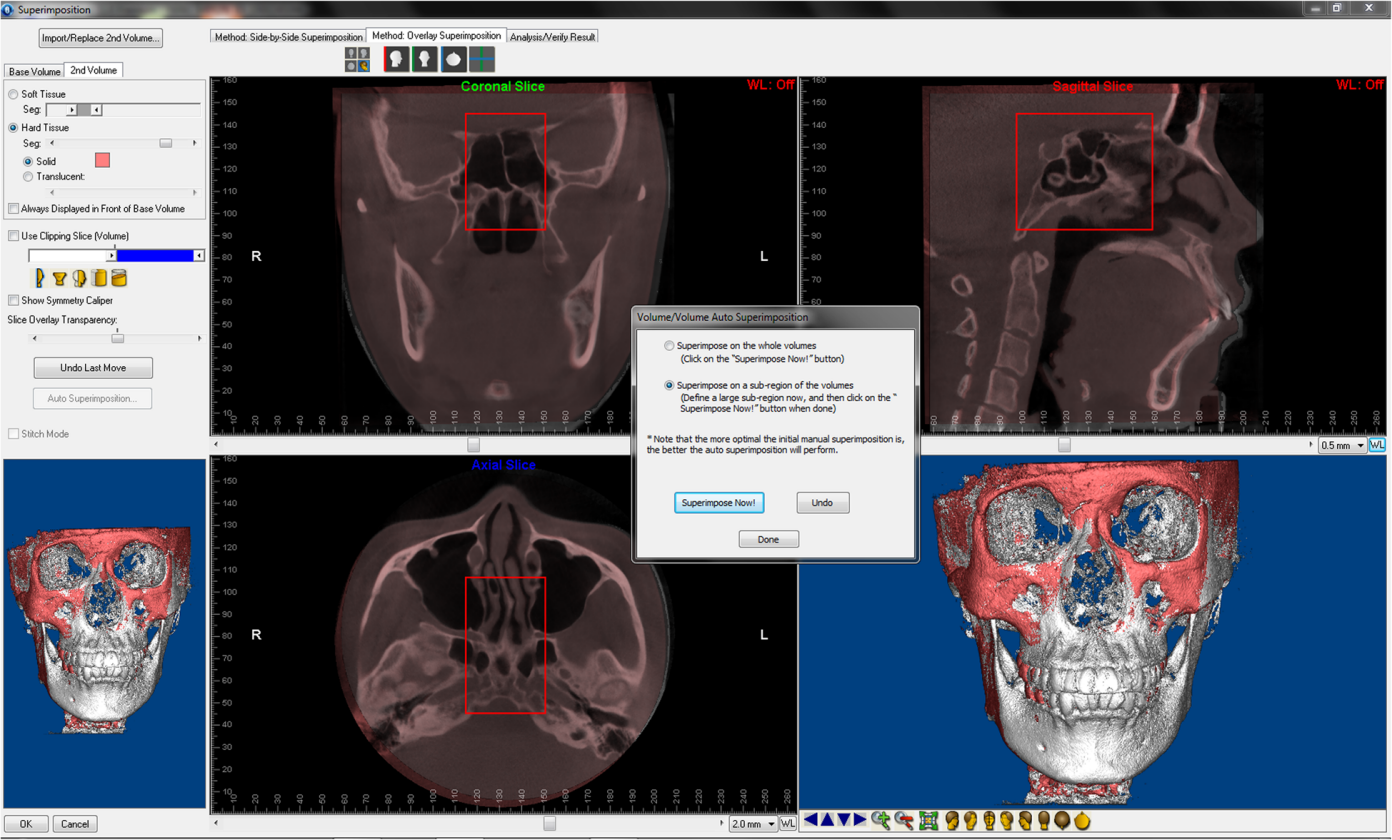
Superimposition of pre- and posttreatment CBCT scans based on the frontal base area (red subregion)
